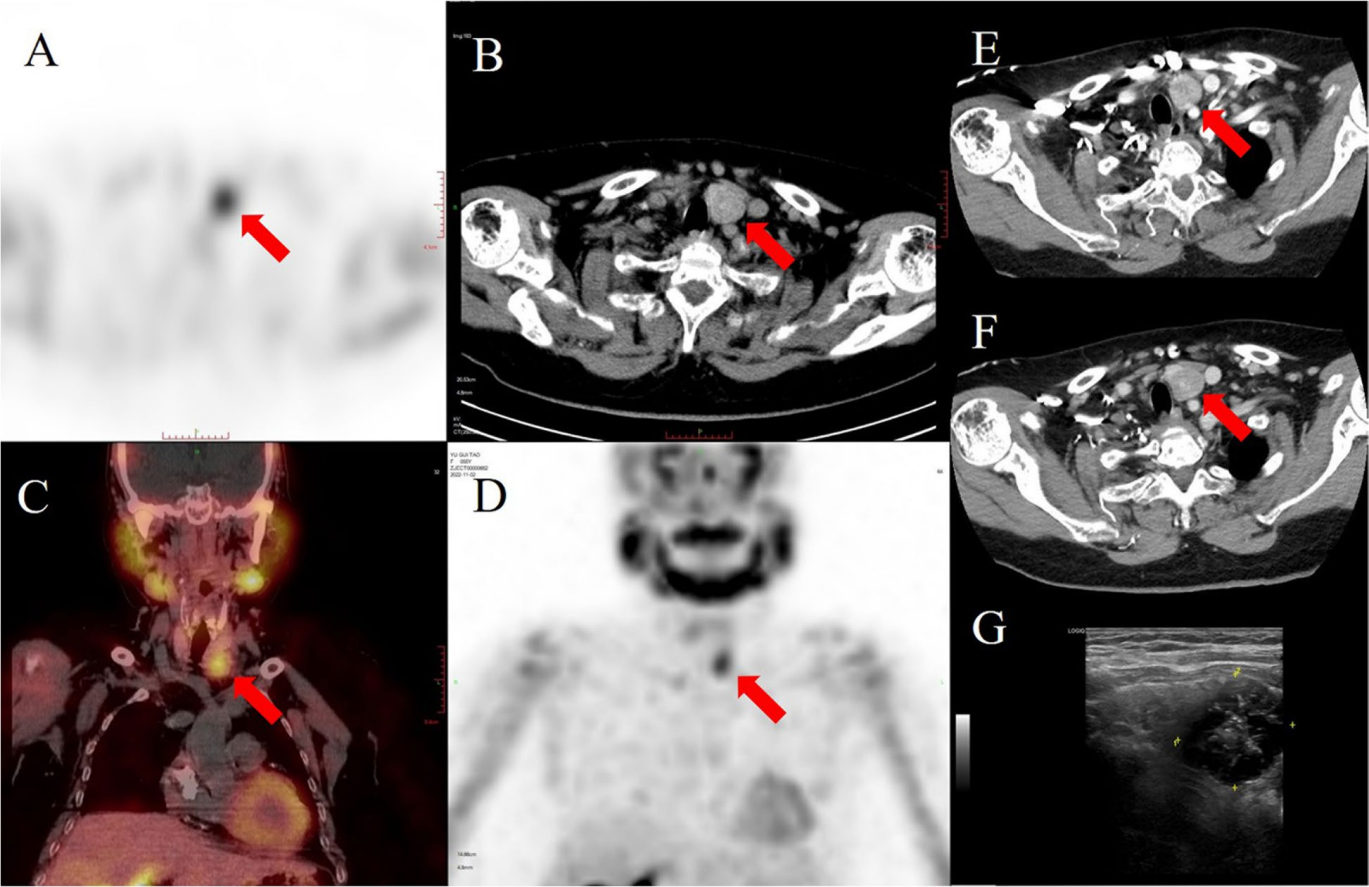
The 99mTc-MIBI SPECT/CT scan shows focused tracer accumulation with a low-density nodule located inferior to the left thyroid gland (red arrows: A SPECT, B axial image, C coronal SPECT/CT fusion image, D coronal SPECT image). Contrast-enhanced CT scan reveals arterial-phase (D) and delayed-phase (E) axial images of a moderately enhanced parathyroid nodule (red arrows) located inferior to the left thyroid gland. Ultrasonographic image of a hypoechoic nodule with irregular margins and calcareous composition also in the left inferior of the thyroid gland (G)
